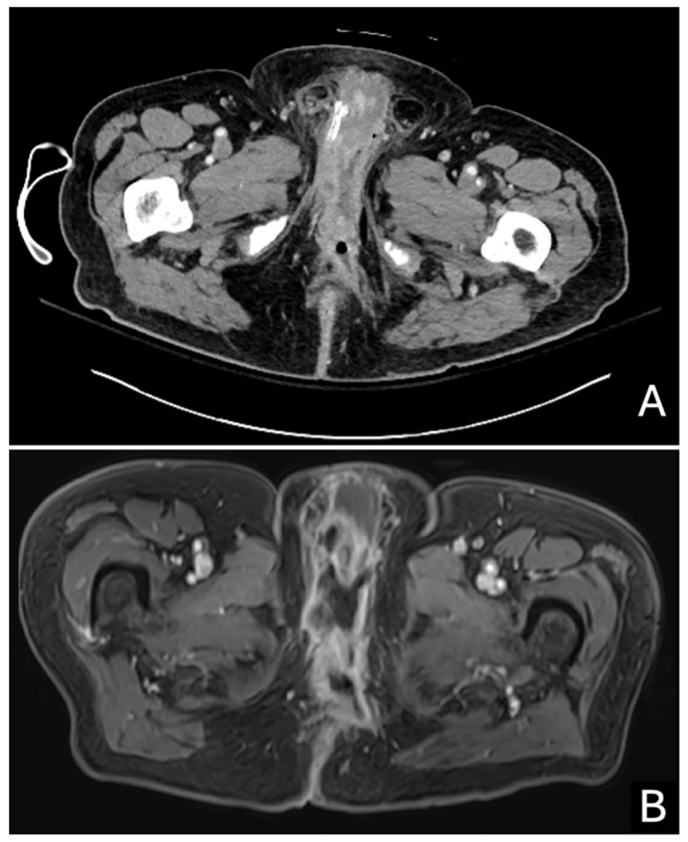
Imaging of the fluid collections. A) CT of scrotal fluid extending to the perineum, measuring 2.2 × 4.6 cm. B) MRI of fluid collection in perirectal space extending to penile base.
